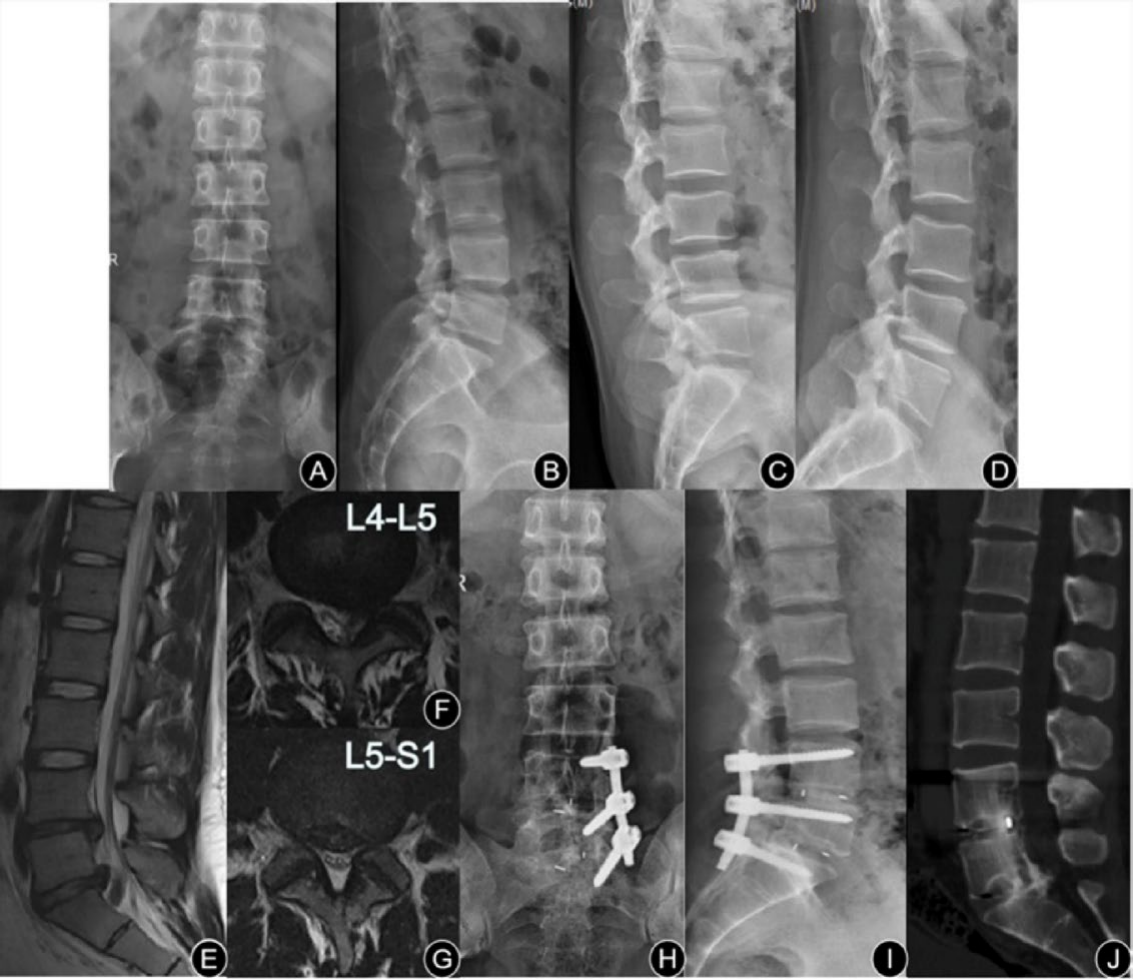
Unilateral pedicle screw fixation (UPSF) combined with intervertebral fusion (IF) to treat two-level lumbar degenerative disease. Male, 39 years old, complaining with lower back pain and radiating pain, numbness of both legs for half a year, and the left is more serious. Preoperative diagnosis was L4-S1 lumbar disc herniation (LDH). (A)–(D) Anteroposterior, lateral, extension and flexion position plain indicated no instability and olisthy. (E) and (G) Preoperative MRI showed L4-S1 LDH; (H) and (I) Anteroposterior and lateral radiograph at 4 years after-surgery indicated that the location of internal fixation was good; (J) CT at 4 years after-surgery showed IF was excellent.
